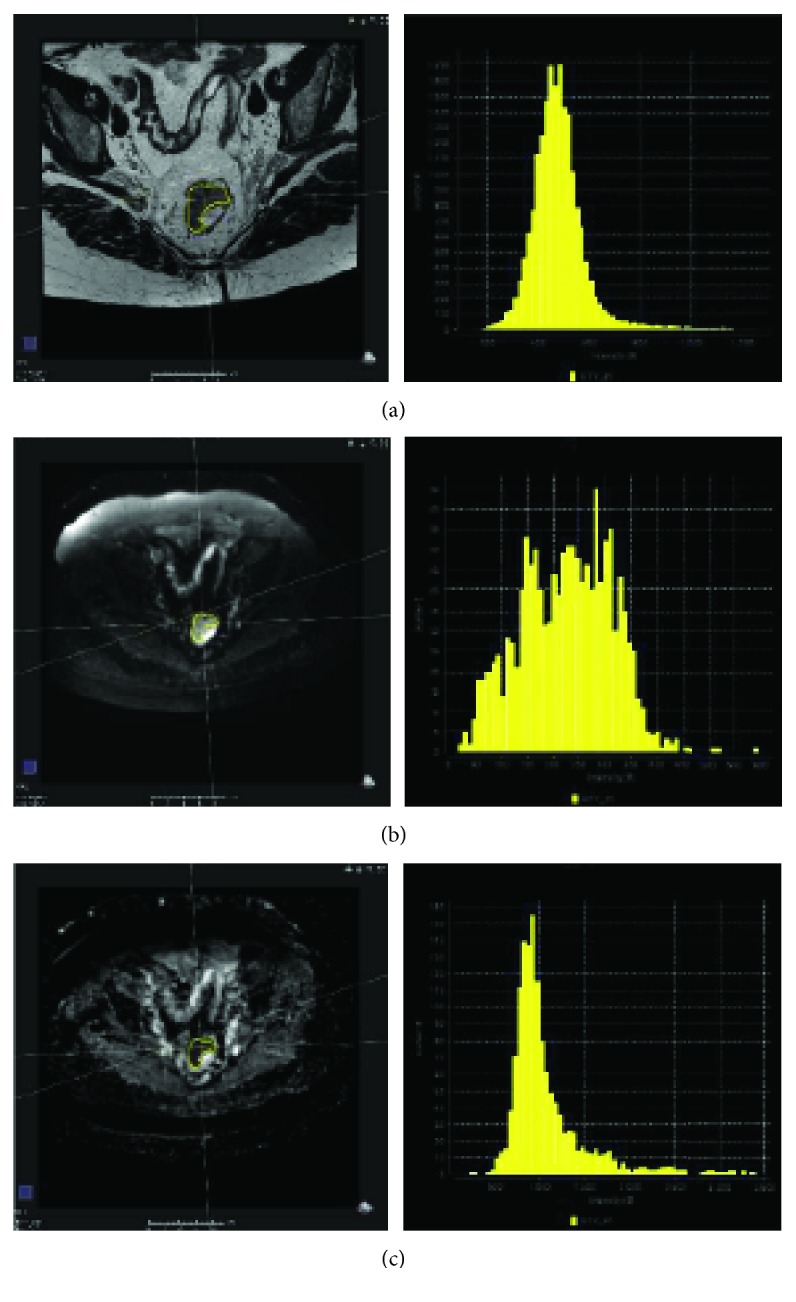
The gross tumor volume (GTV) was evaluated at baseline by MRI and contoured on T2 (a), DWI (b), and ADC (c) sequences.
